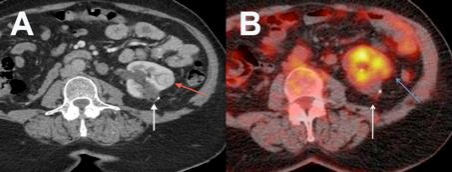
Imaging at 15 months follow-up of patient number 5. A, Intravenous contrast-enhanced CT scan in patient number 5 showing a non-enhancing zone (white arrow) at the side of the renal lesion treated with LCA. A second solid lesion with clear contrast enhancement (red arrow) was noticed. B, At 18F-FDG PET-CT, the ablation zone showed no metabolic FDG uptake (white arrow). The blue arrow indicates the region of the metachronous RCC with FDG uptake (SUV 3.1).
